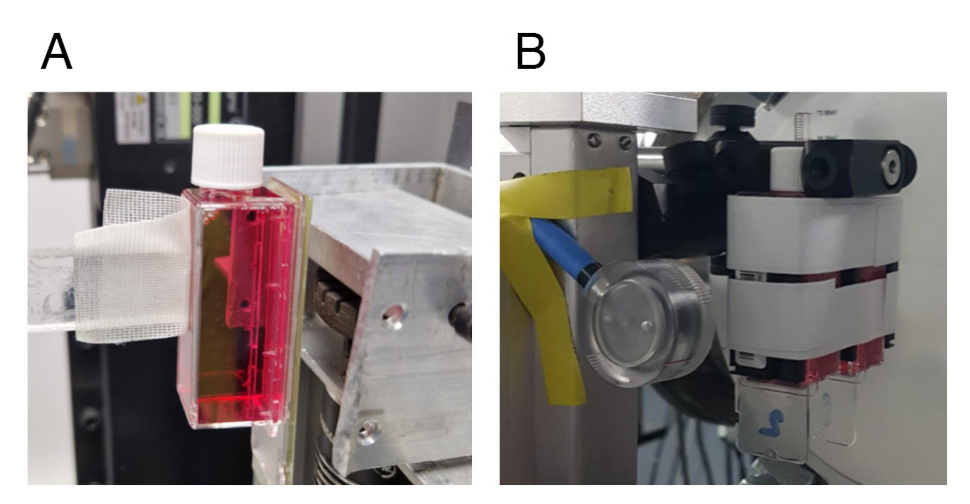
Photographic image exemplifying the mounting of media-filled SlideFlasks onto the support before targeting the sample with the X-ray beam (A) or LPA electrons (B)
